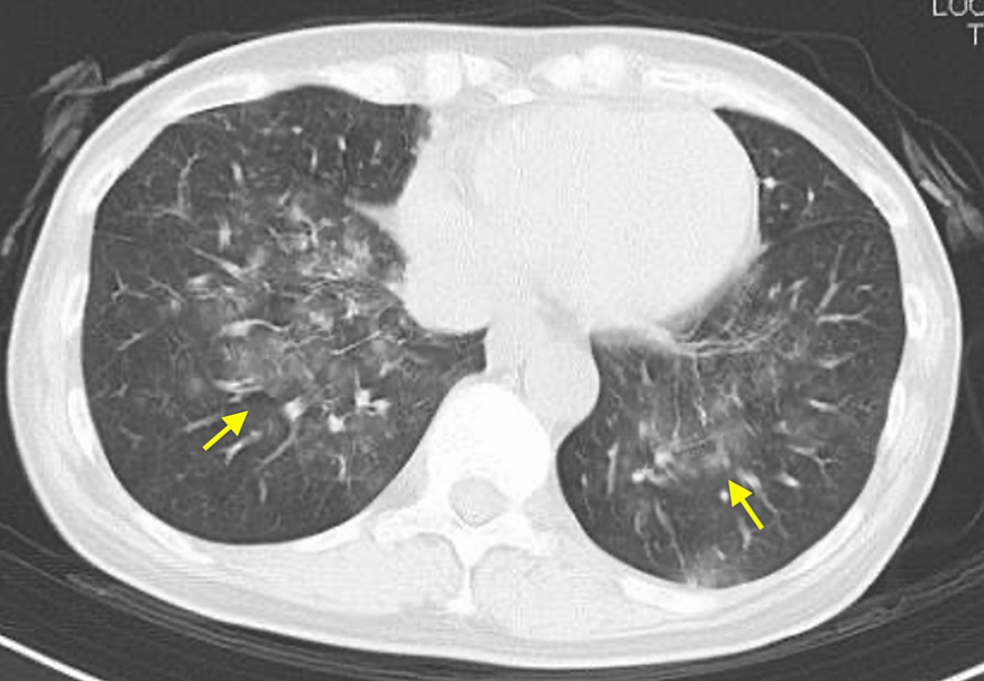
Computed tomography of the chest. Arrows indicate the spread of reticular shadows to the bilateral lungs.
